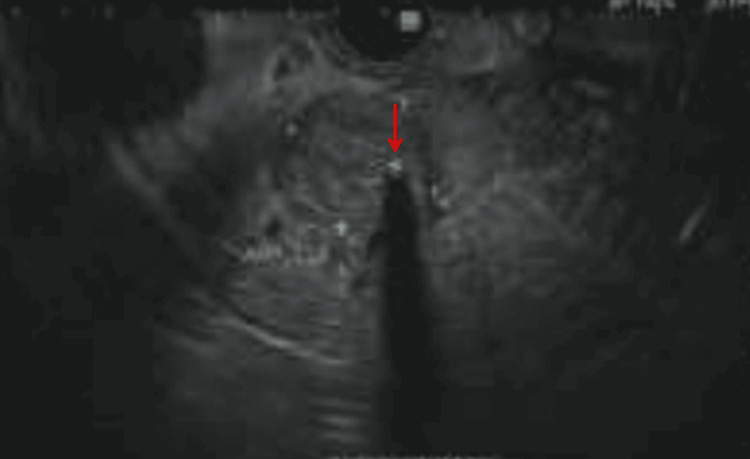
On endoscopic ultrasound, a hypoechoic oval mass (red arrow) with post-acoustic shadowing was identified endosonographically in the ampulla, measuring 16 mm by 15 mm in cross-sectional diameter.
